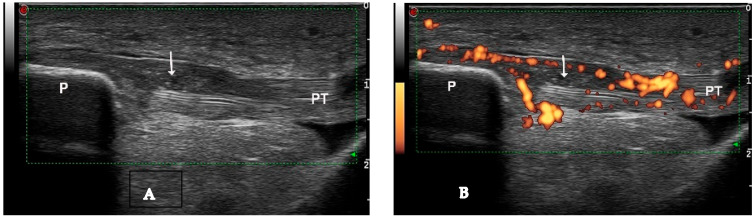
Intratendinous soft tophus visible in longitudinal view of the proximal insertion of the patellar tendon ((A)—grayscale, (B)—Power Doppler mode). P—patella, PT—patellar tendon, arrow—intratendinous soft tophus.
